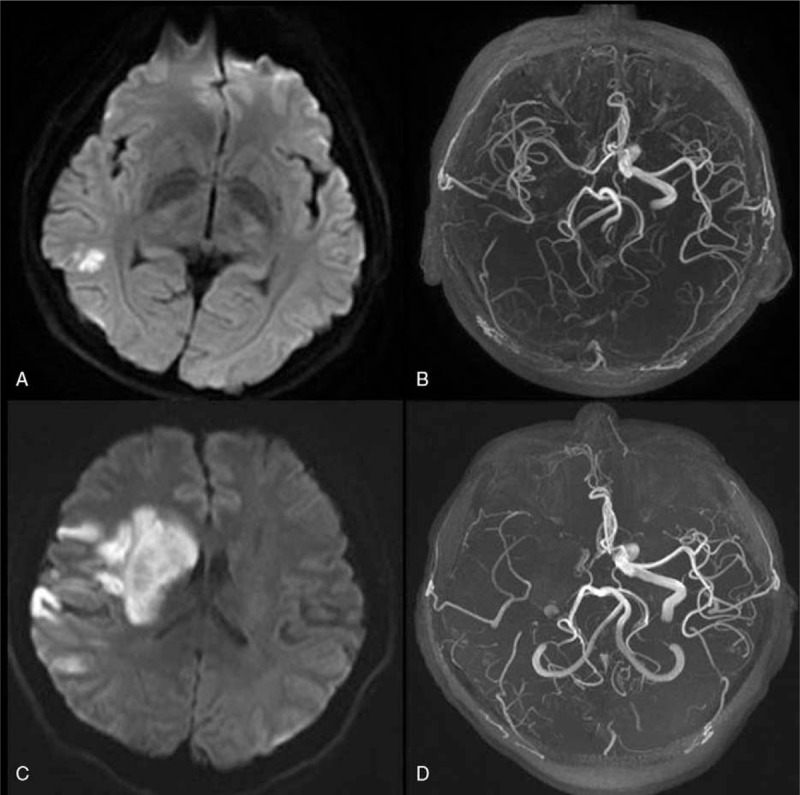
Imaging examination of the brain and cerebral vessels. DWI (A) showed increased signal intensity, indicating acute infarction in the right postwatershed area. MRA (B) demonstrated severe stenosis versus occlusion of the right internal carotid artery. Repeat DWI and MRA (C, D) revealed additional infarction in the right basal ganglia and the occlusion of the right middle cerebral artery. DWI = diffusion weighted imaging, MRA = magnetic resonance angiography.
